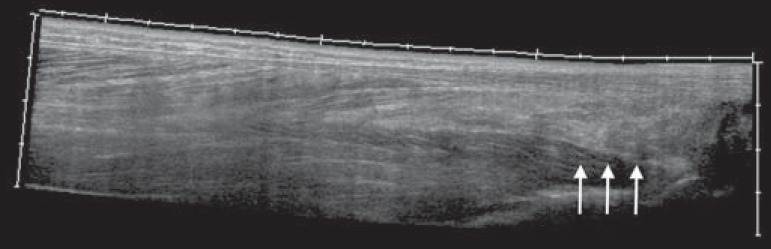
Sagittal ultrasound image of the posterior calf region, performed with a
